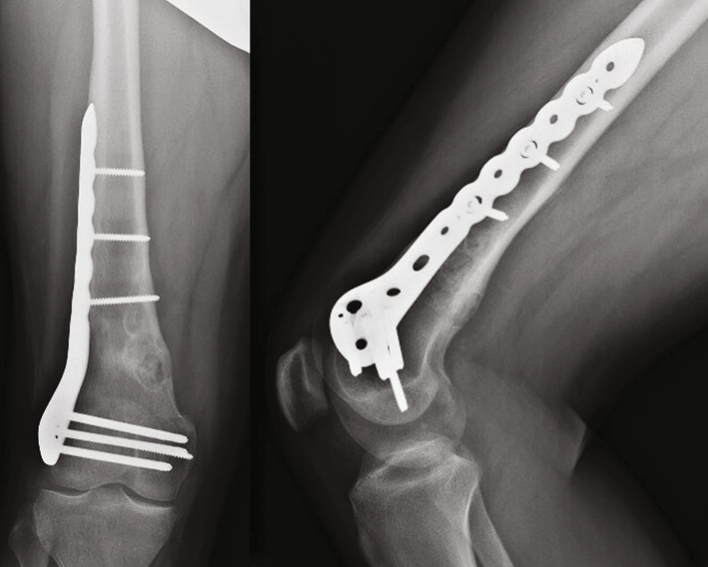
AP and lateral plain radiographs of the distal femur obtained at the final clinic appointment, 6 weeks postoperatively, following diagnostic arthroscopy, curettage, and bone grafting with completely healed fracture.
